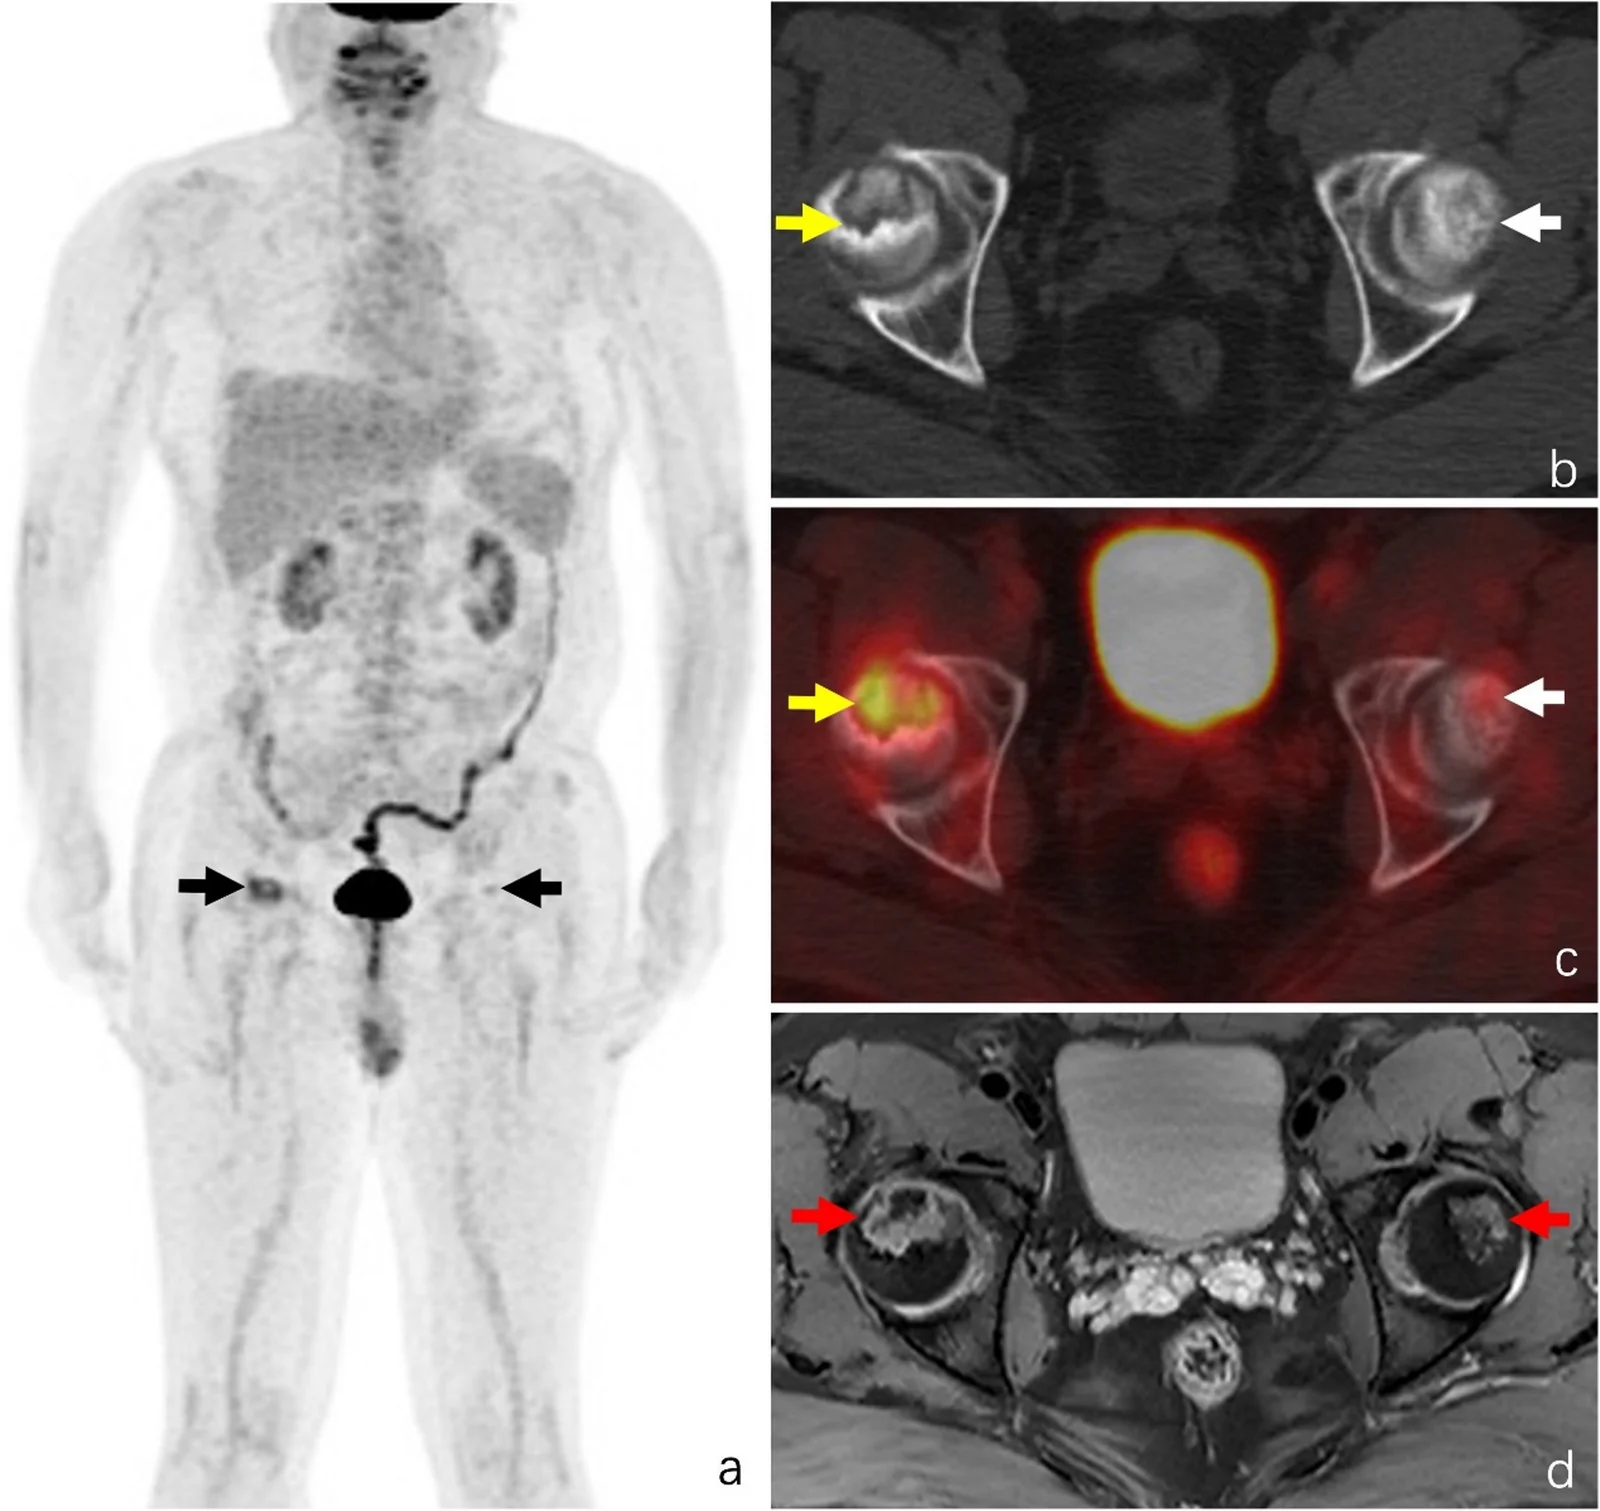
Osteonecrosis of the femoral heads. PET/CT and MRI images from a 44-year-old male (No. 11) with mantle cell lymphoma. The maximum intensity projection image of PET (a) shows increased ^18^F-FDG uptake (black arrows) in the regions of the bilateral femoral heads. The CT image (b) demonstrates osteolytic area with internal sequestrum, peripheral osteosclerosis, and subchondral fracture at the right femoral head (yellow arrow), as well as high density at the left femoral head (white arrow). The PET/CT fused image (c) shows increased ^18^F-FDG uptake at the bilateral femoral heads with SUV_max_ 4.41 (yellow arrow) and 2.23 (white arrow), respectively. An MRI scan performed three days after the PET/CT shows geographic abnormal signals at the bilateral femoral heads on the fat-saturated proton density-weighted image (red arrows in d). According to the modified 2019 version of the Association Research Circulation Osseous international classification of osteonecrosis staging system, the osteonecrosis in the right femoral head is classified as stage 3
